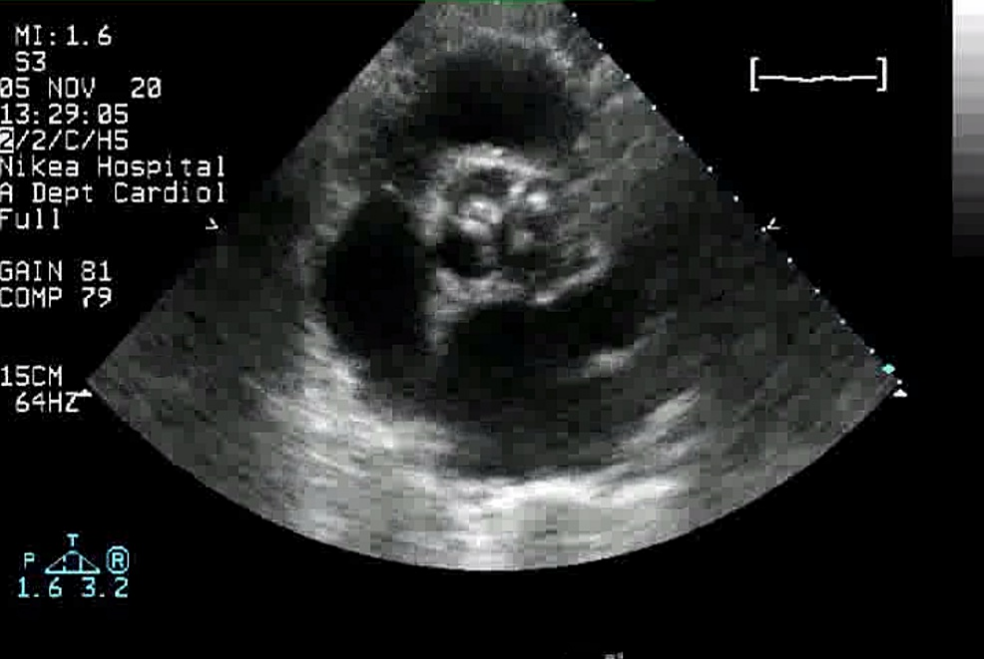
TTE depicting the abscess in the patient’s native aortic valve and surrounding tissue. TTE: transthoracic echocardiogram
